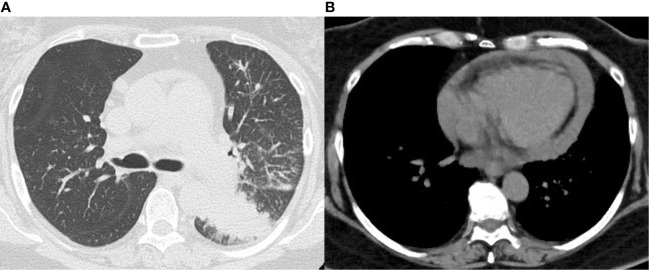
Chest computed tomography on admission. (A) Lung window shows a mass shadow in the lower lobe of the left lung. (B) Mediastinal window shows pericardial effusion.
